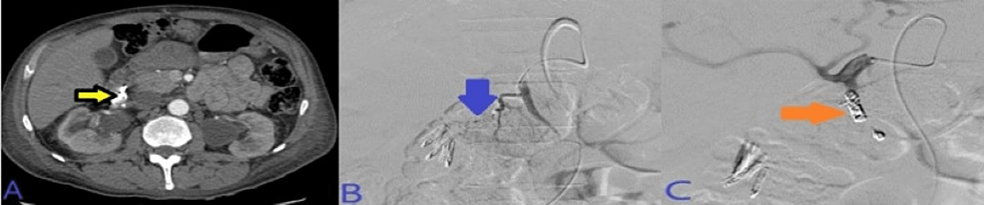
A: CT angiogram of the abdomen demonstrated hyperdense materials/blood products within the second portion of the duodenum adjacent to multiple endoscopic clips. B: Super-selective GDA angiograms using microcatheter systems demonstrated duodenal culprit bleeding with many irregular tortuous arteriolar feeders from superior anterior duodenal arterial branches of the GDA, uncontrolled by adjacent clips placed earlier by endoscopy. C: Post-embolization selective common hepatic angiograms demonstrated no evidence of active bleeding signs, and complete resolution of the above findings after five coils' deployment in “sandwiching” appropriate positions, beyond the takeoff of main GDA and through the proximal aspect of right gastroepiploic artery, with adequate hepatic arterial inflow. CT: computed tomography; GDA: gastroduodenal artery.
